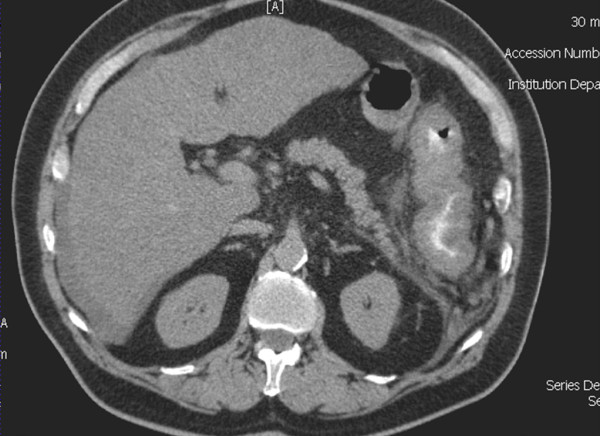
CT scan of case 2 showing again extensive inflammation of the entire colon (pancolitis), with oedema and thickening of the large intestine wall.
